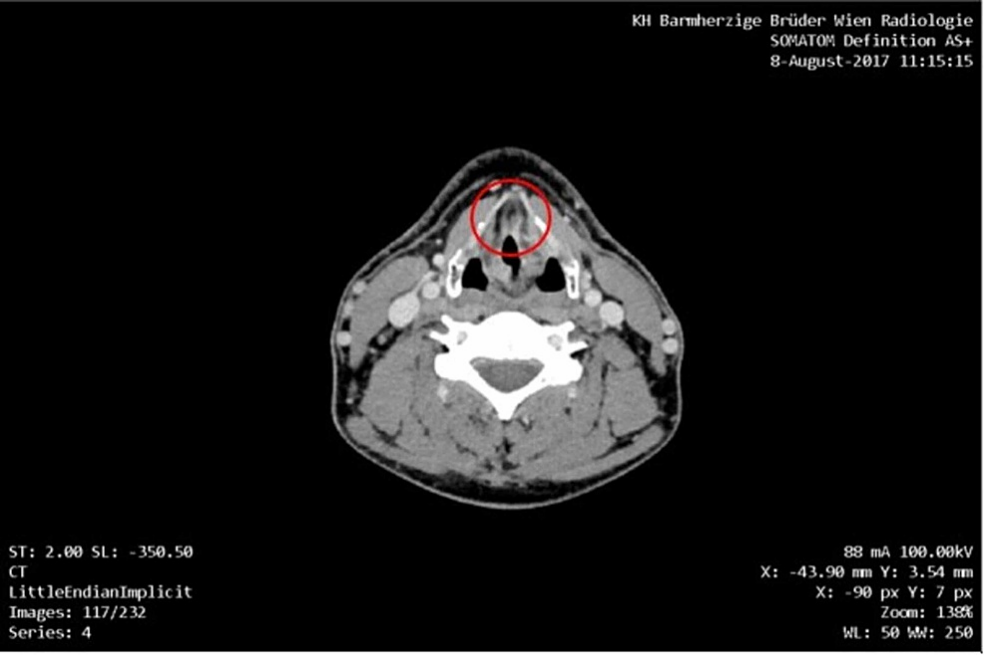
CT scan with contrast agent from August 2017. Hypodense formation (11 mm x 8 mm x 13 mm) at the commissura anterior of the vocal cord on the left, showing the recurrent tumor. No suspect lymph nodes, no lung metastases in the upper thorax, and no osseus metastases.
